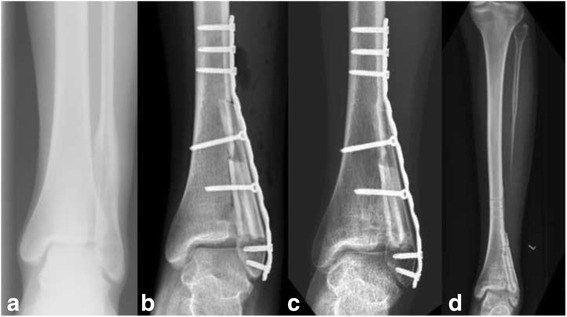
Ewing’s sarcoma of the distal fibula in a 15-year-old female national squad triathlete a After wide resection of the tumour under preservation of the malleolar tip, the distal fibula was reconstructed with a non-vascularised fibula strut by performing a tibio-fibular synostosis b After complete integration and bony consolidation of the fibula graft c removal of the plate as well as the screws was planned as the patient felt bothered during sports activities d Partial remodelling of the fibula with ossifications along the periosteum was seen on conventional X-rays of this patient d
